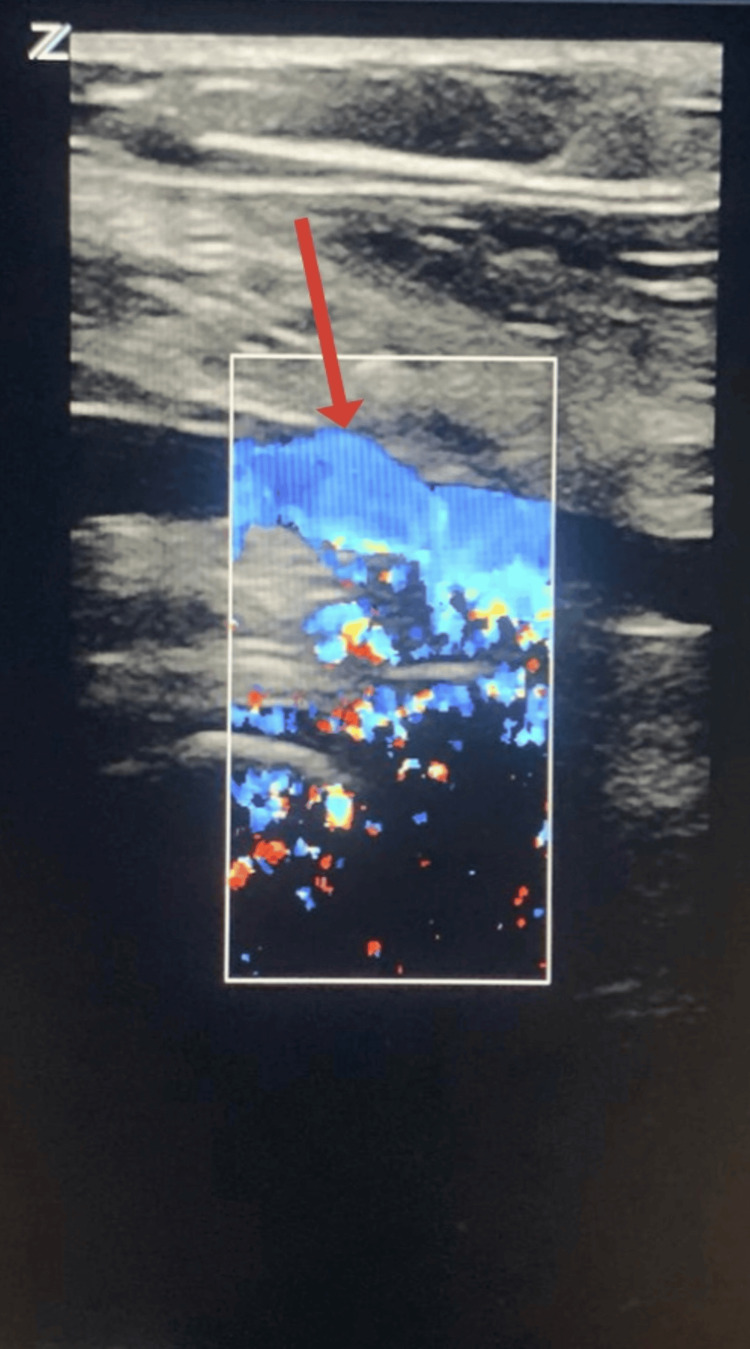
Ultrasound duplex scan of the venous repair site The red arrow shows the flow in the arterial interposition graft used to repair the popliteal vein.
